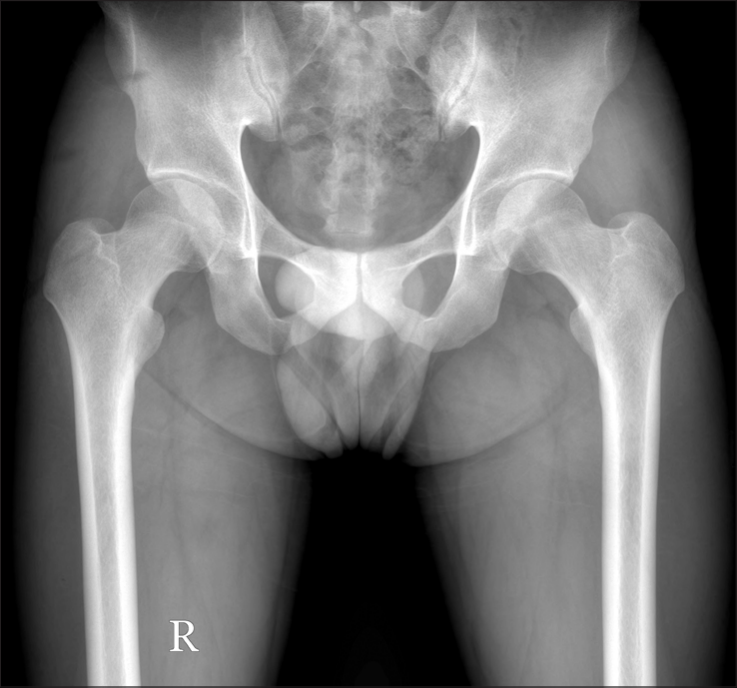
Both hip anteroposterior radiograph of a 25-year old male who suffered from left hip joint pain for 3 years shows a positive crossover sign in both hip areas.
